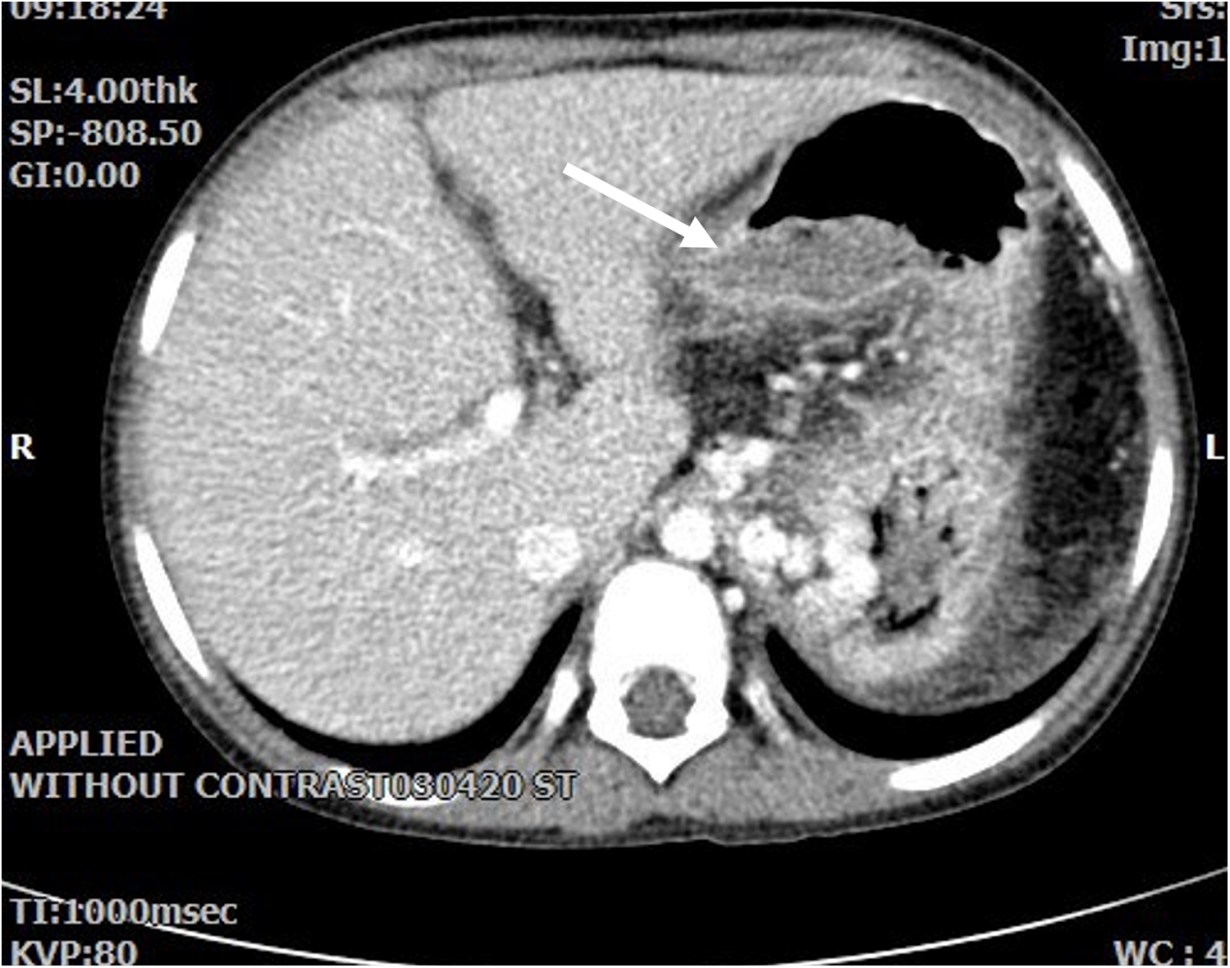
Axial abdominal CT with contrast show evidence of previous splenectomy and extensive gastric varices. Dilated varicose veins protrude intraluminally in fundus with a large gastric intraluminal clot related to recent hemorrhage.
